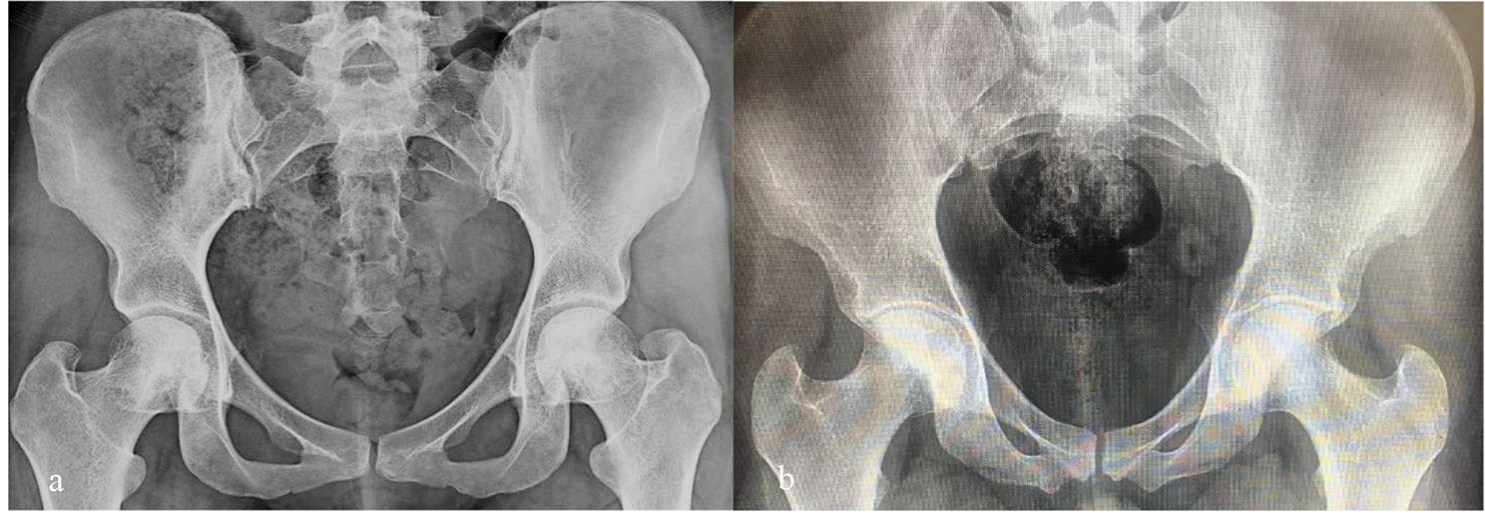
Preoperative (a) and (b) postoperative Radiography
